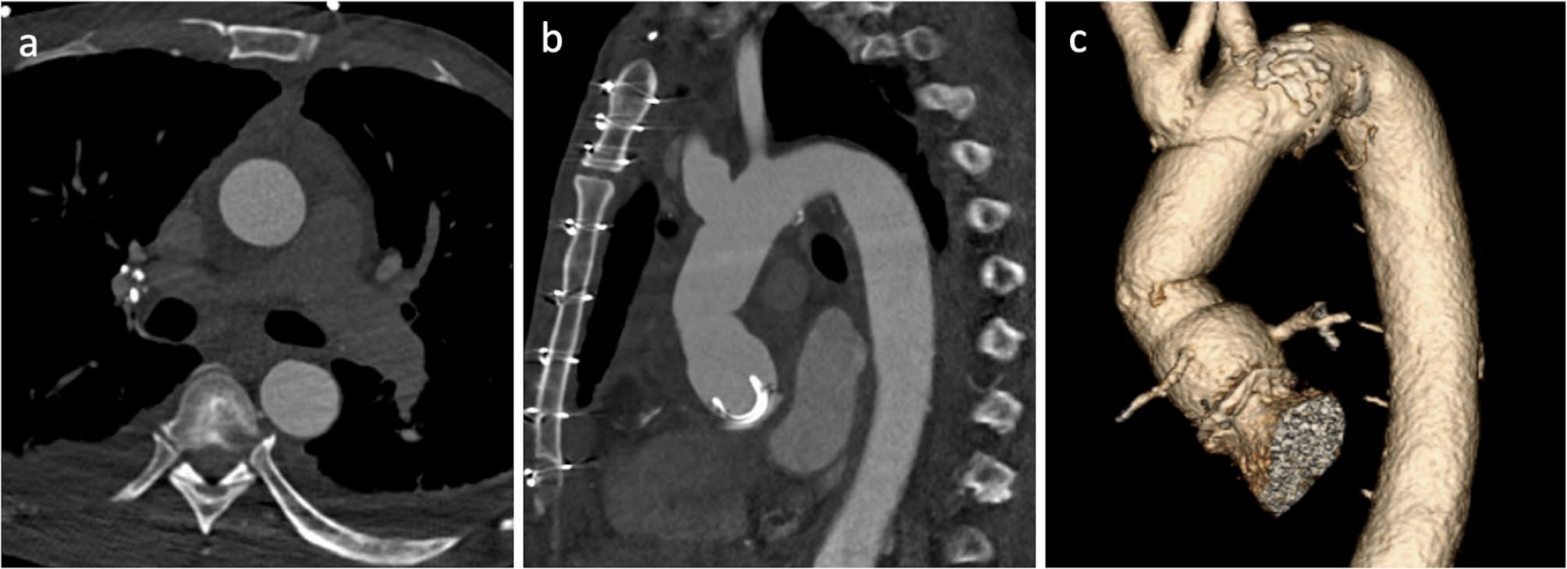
(a) Transverse view of postoperative computed tomography shows reconstructed ascending aorta. (b) Sagittal view of postoperative computed tomography shows reconstructed aortic root and ascending aorta. (c) Reconstructive computed tomography angiogram shows reconstructed aortic root and ascending aorta with decent proximal coronary artery enhancement
